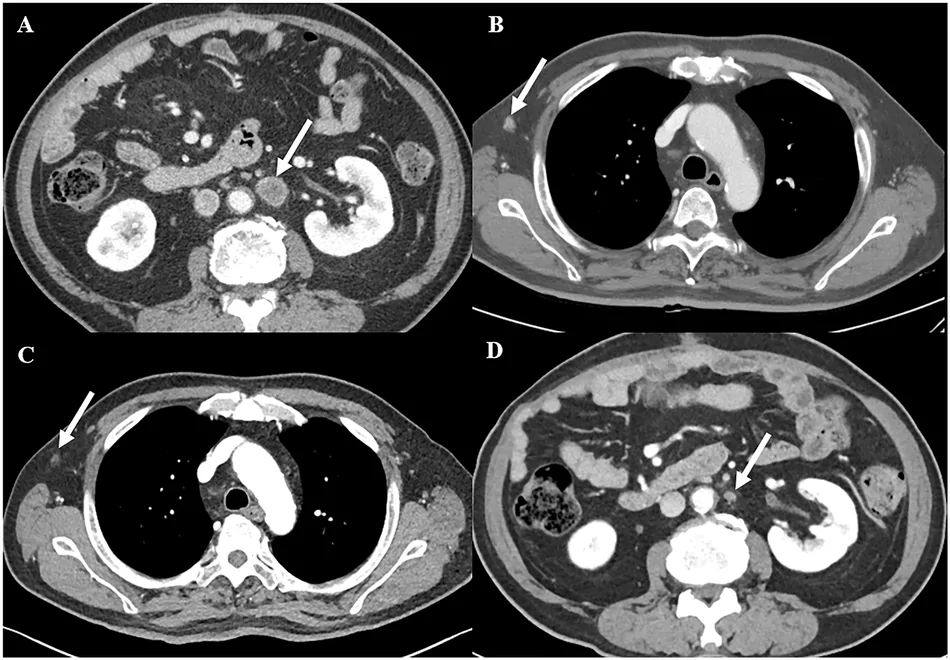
A 62-year-old man with recurrent intrahepatic cholangiocarcinoma following surgery. A Contrast-enhanced axial portal venous phase CT obtained 18 months after right hemihepatectomy demonstrates a 2-cm enlarged lymph node in the left para-aortic region (arrow), consistent with retroperitoneal lymph node metastasis. The patient subsequently received treatment with durvalumab, gemcitabine, cisplatin, and tremelimumab. B Follow-up contrast-enhanced axial chest CT at 2 months after initiating treatment including durvalumab reveals mild enlargement of a right axillary lymph node (arrow). C Follow-up contrast-enhanced axial chest CT at 4 months demonstrates a spontaneous decrease in the size of the previously enlarged right axillary lymph node (arrow). D Concurrent contrast-enhanced axial portal venous phase CT at 4 months shows a marked reduction in the size of the metastatic left para-aortic lymph node (arrow), consistent with a partial response to treatment. The patient ultimately died of disease progression 34 months after initiation of durvalumab-based therapy
